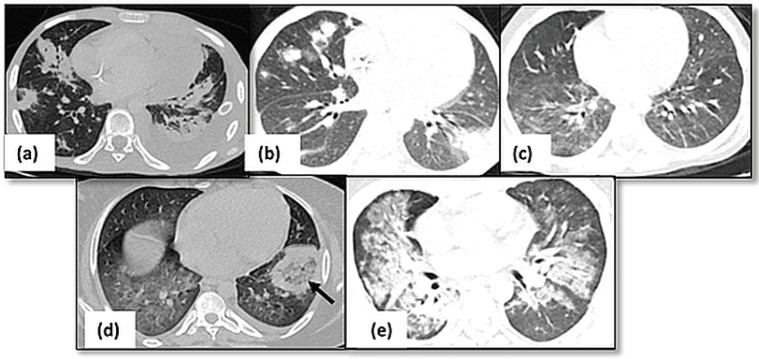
Pulmonary infections. (a): Axial HRCT image showing multiple consolidations in bilateral lungs with left sided pleural effusion suggestive of bacterial pneumonia. (b): Fungal infection with multiple nodules having surrounding ground glass opacity in bilateral lungs. (c): Axial HRCT image of a patient with viral pneumonia showing patchy areas of ground glass opacity in bilateral lungs. (d): Axial HRCT image showing presence of consolidation with central ground glass opacity (arrow) in left lower lobe suggestive of mucormycosis. (e): Consolidation and ground glass opacity seen in perihilar and peribronchovascular locations s/o PCP pneumonia.
